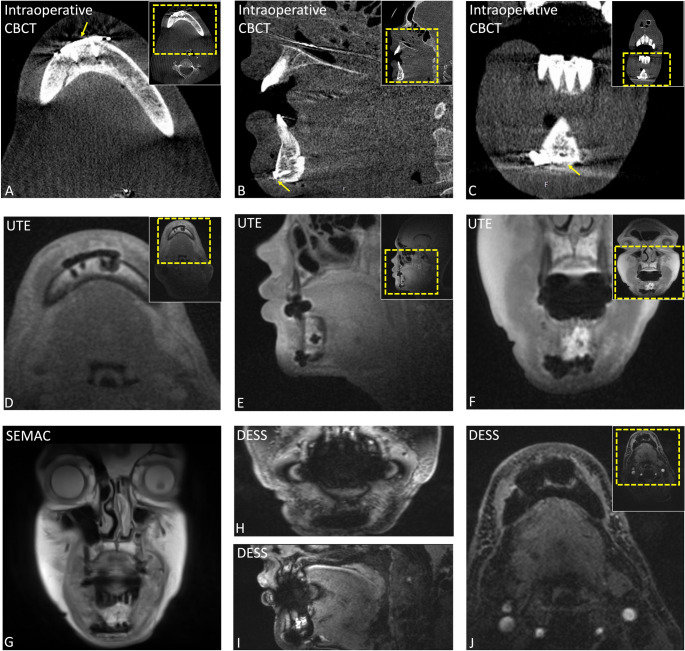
Management of a paramedian mandibular fracture using osteosynthesis plates and lag screws. Intraoperative CBCT images (A: coronal, B: sagittal, C: axial; arrows indicate osteosynthesis material). MRI-based visualization with UTE (D: coronal, E: sagittal, F: axial) and SEMAC (G) demonstrates signal voids caused by the osteosynthesis material, whereas fracture reduction and osteosynthesis material are poorly assessable on DESS images (H-J). CBCT: Cone-beam computed tomography; UTE: 3D ultrashort echo time; SEMAC: Slice-encoding for metal artifact correction sequence; DESS: 3D double-echo steady-state
